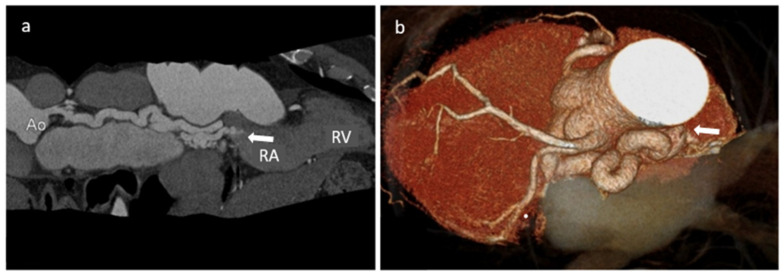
A 66-year-old male patient with a left main coronary artery to right atrium (RA) fistula (arrow) in computed tomography curved planar reconstruction (a), volume-rendering (b) images. Ao: aorta; RV: right ventricle.
